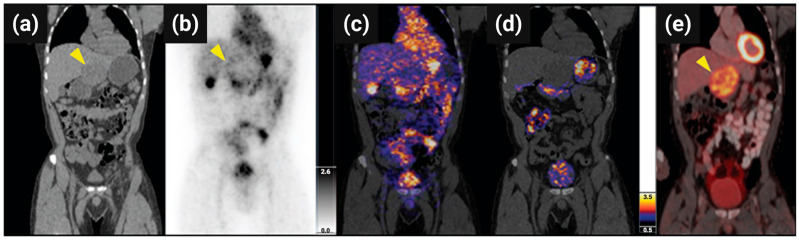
Whole-body PET-CT imaging of a patient with anorectal mucosal melanoma and a known left hepatic lobe metastasis following intravenous administration of ^124^I-cRGDY–PEG–C dots. (a) Reformatted coronal CT acquired 4 h post-injection demonstrating a hypodense lesion in the inferior left hepatic lobe (yellow arrowhead), (b) coronal PET image at 4 h post-injection showing particle uptake circumscribing the tumor margin (yellow arrowhead), as well as activity in the bladder, gastrointestinal tract, gallbladder, and heart, (c) co-registered PET-CT at 4 h, confirming particle localization to the tumor margin, (d) co-registered PET-CT at 24 h, indicating clearance of particle activity from the tumor, and (e) corresponding ^18^F-FDG PET-CT image, revealing a hypermetabolic tumor border in the hepatic metastasis (yellow arrowhead), reflecting glucose metabolism. Reproduced with permission [140].
